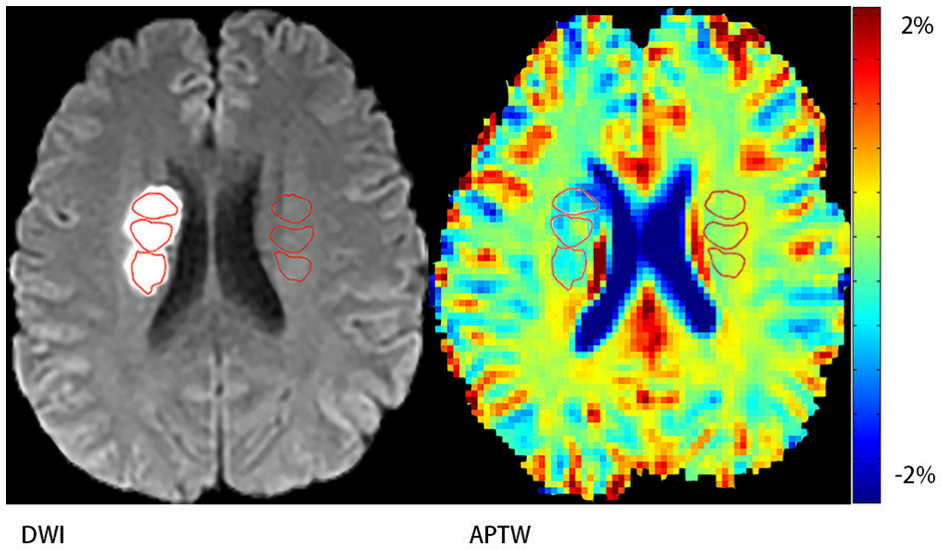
Demonstration of regions of interest (ROIs). Three ROIs on the stroke hemisphere within the stroke area defined by DWI and another three ones on the contralateral hemisphere.
